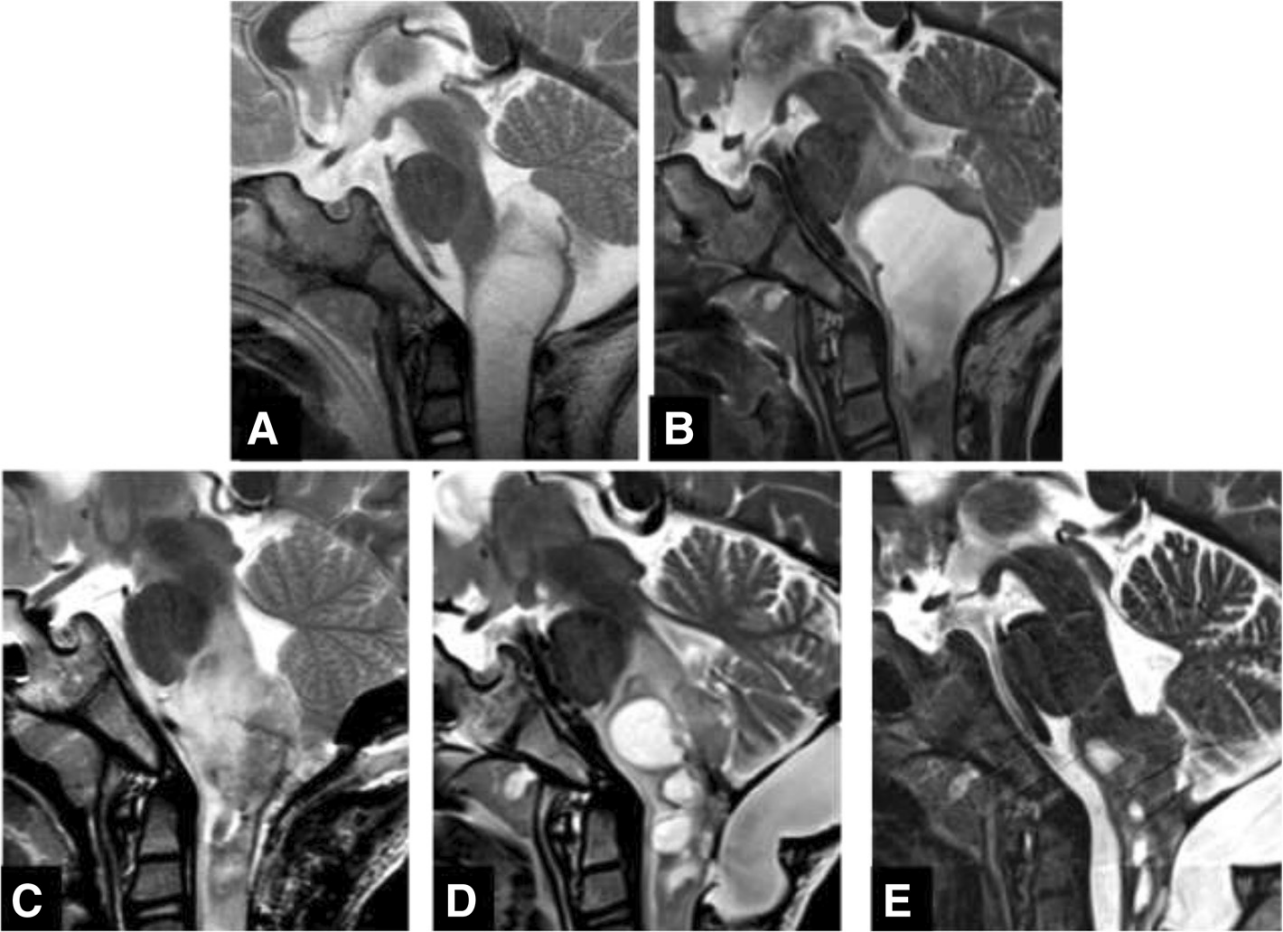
Serial MRI features of the lesion. Sagittal T2 weighted images show, at onset, a bulky mass extending from medulla into cervical spinal cord, dislocating the pons, the floor of the IV ventricle, the cerebellar vermis and tonsils (A); increased size of both cystic and solid component of the lesion after surgical decompression and chemotherapy (B); a new disease progression three months after second surgery (C); further increase of cystic components (D); a relevant reduction in size of both the solid and the cystic components of the lesion six months after the start of treatment (E).
